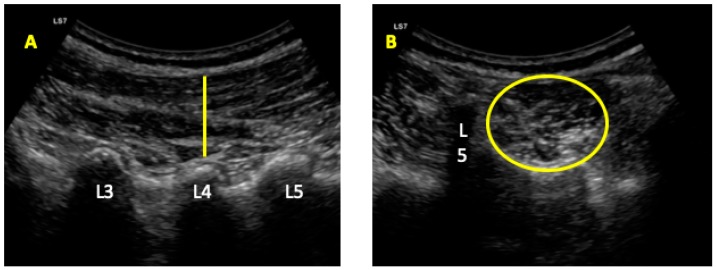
Ultrasound imaging for the multifidus thickness (A) and cross-sectional area (CSA) (B).
